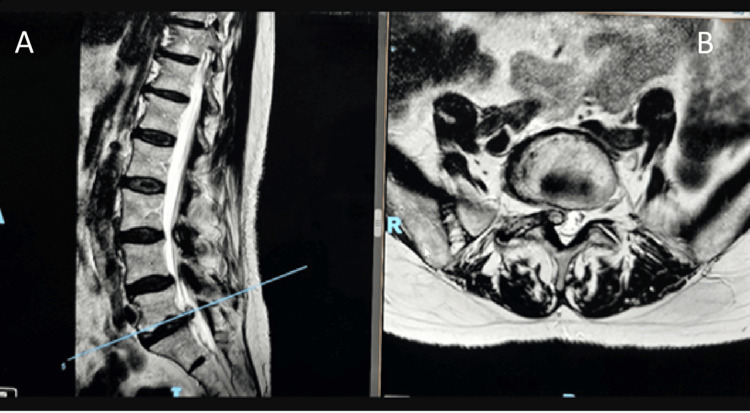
MRI lumbosacral spine Shows a representative MRI lumbar spine from one of our cases, demonstrating at the L5-S1 level a large right paracentral disc extrusion compressing the thecal sac, occupying and effacing the right lateral recess with compression to the transiting nerve root. There is also an associated facet joint cyst. (A) sagittal view; (B) axial view
